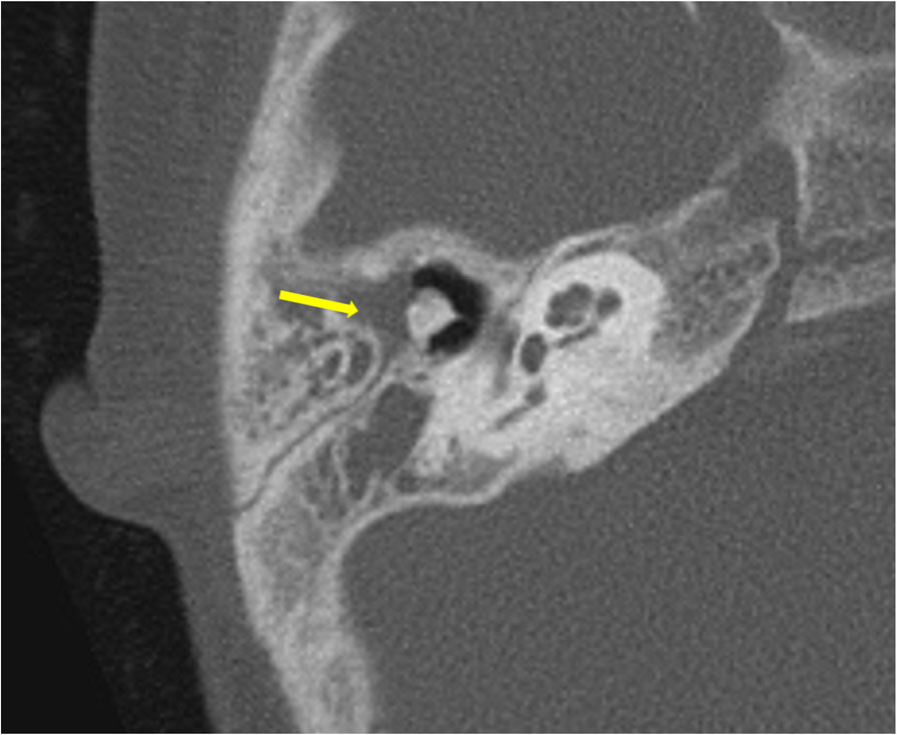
Axial CT image showing non-dependent soft tissue in the Prussack’s space, lateral to ossicles in a patient with pars flaccida cholesteatoma (arrow)
